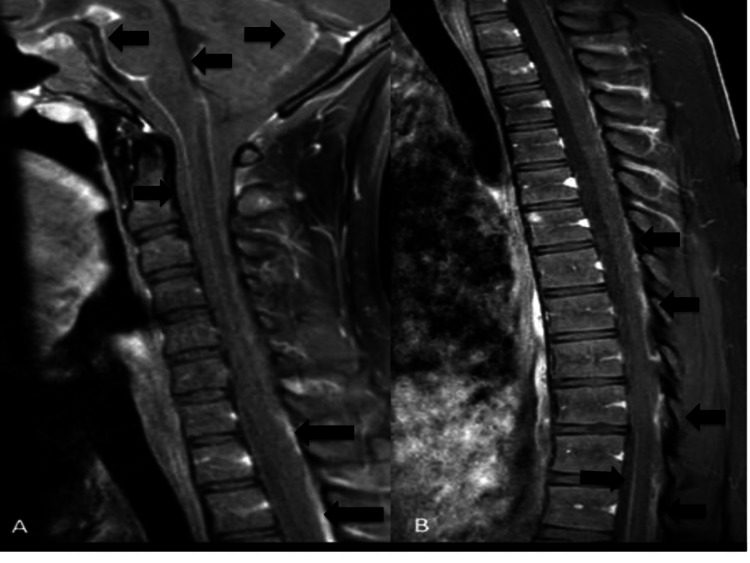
T1-weighted post-contrast MRI of cervical (A) and thoracic (B) spine showing leptomeningeal enhancement
